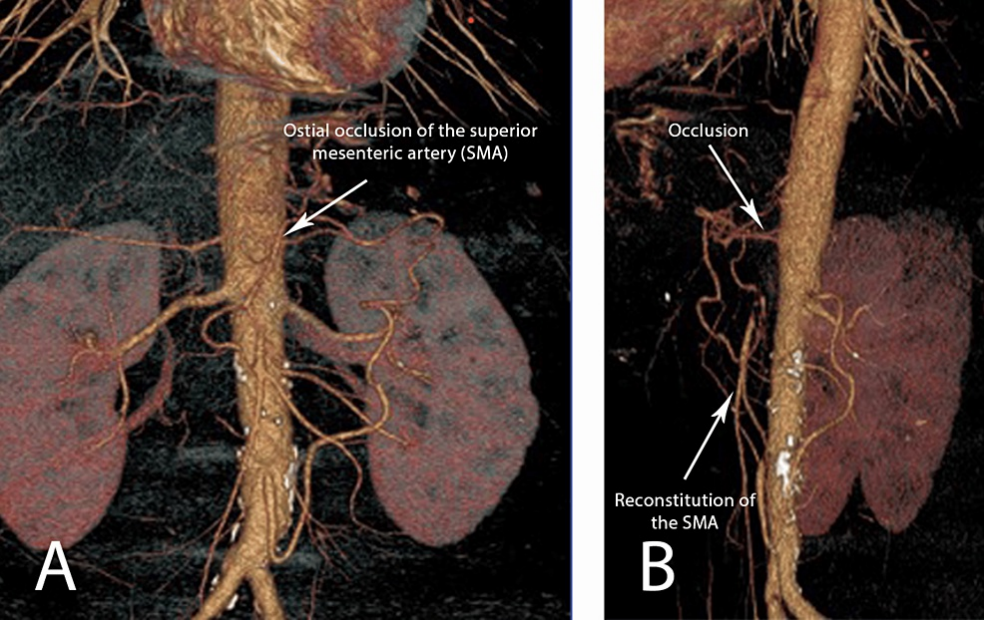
Preoperative CT-Angiogram showing total ostial occlusion of the superior mesenteric artery А-Frontal view; B-Side view; SMA: superior mesenteric artery
